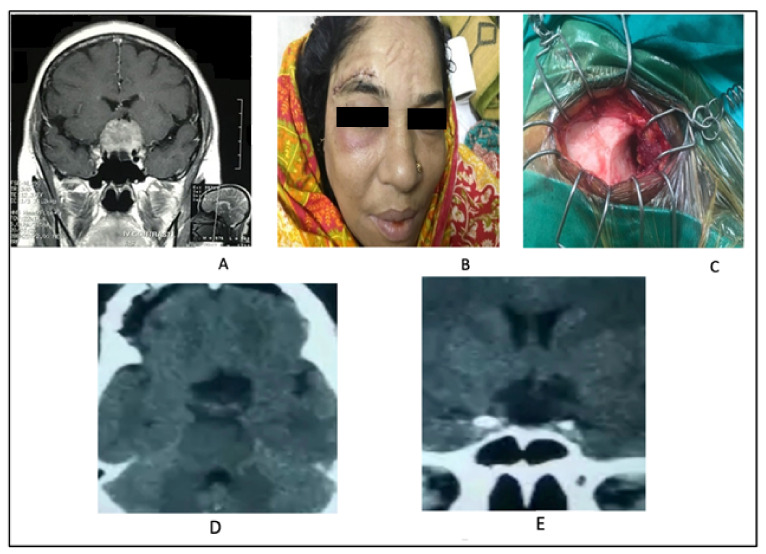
(A) Coronal view MRI with contrast showing a medium-sized TSM. (B) Showing a linear mark of supraciliary keyhole approach. (C) Per-operative picture of supraciliary keyhole exposure showing the frontal bone. (D) Post-operative CT scan of T.S meningioma showing no residual tumor in axial view. (E) No residual tumor in coronal view in post-operative CT scan.
